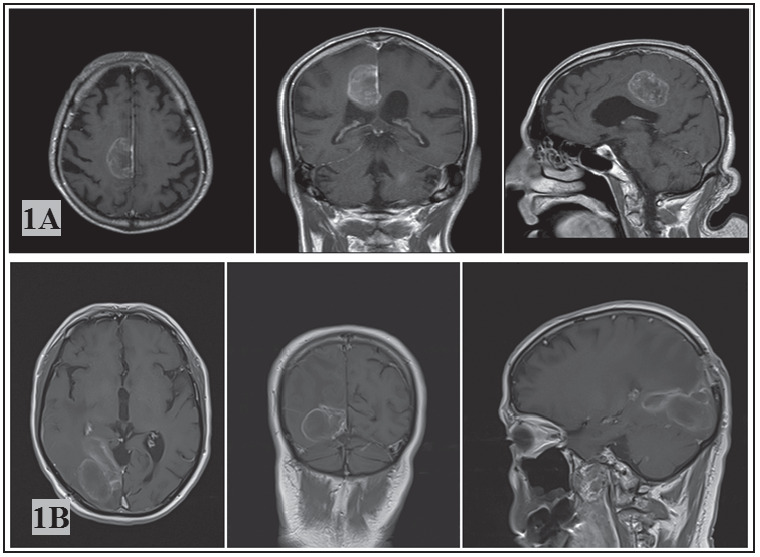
Cranial MR images of patients with intracranial solitary tumor: (1A) axial, coronal and sagittal T1 weighted images of MR with gadolinium in patient with glioblastoma; (1B) axial, coronal and sagittal T1 weighted images of MR with gadolinium in patient with brain metastasis
